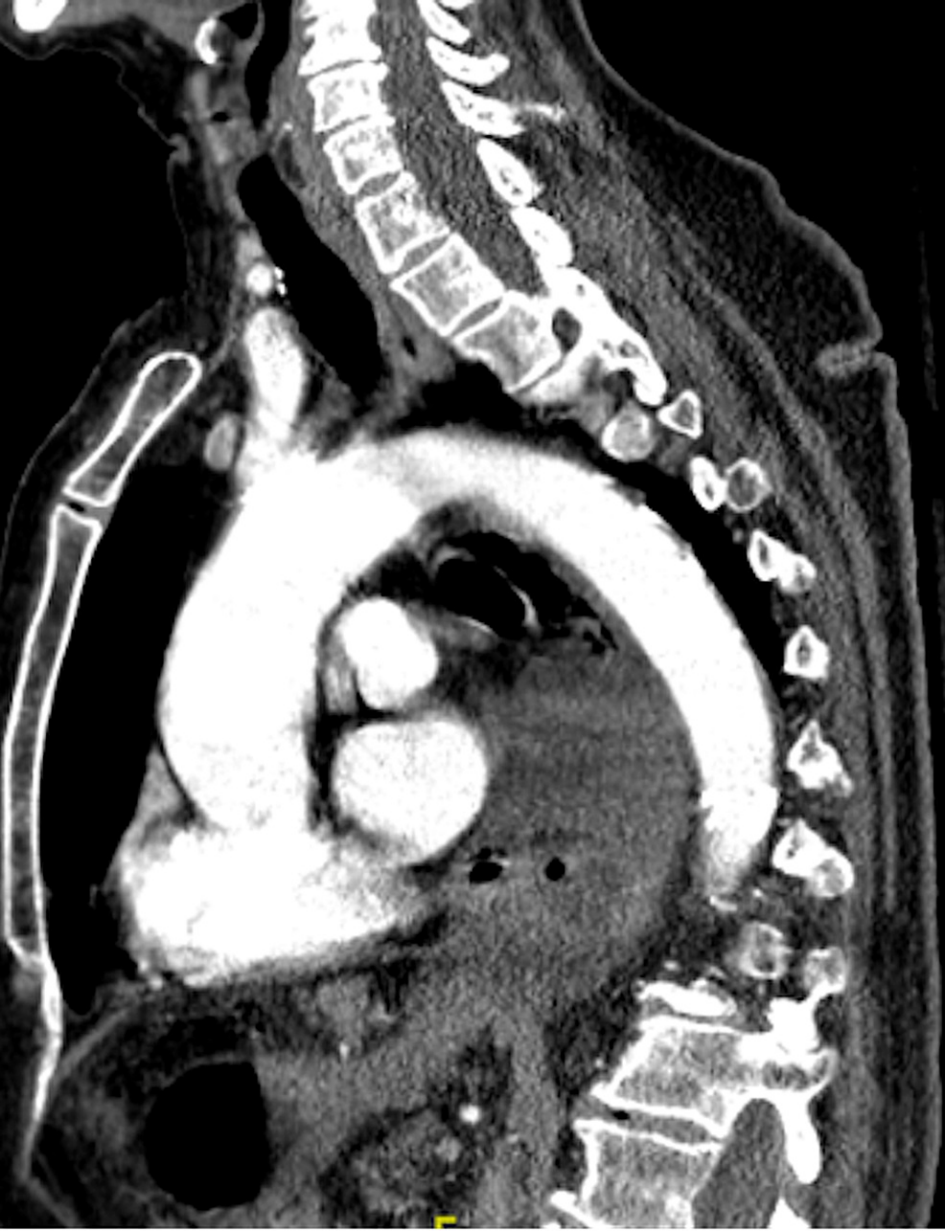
CT with contrast angiography with a sagittal view of a large hiatal hernia with suspected organo-axial volvulus and free fluid surrounding the distal esophagus
